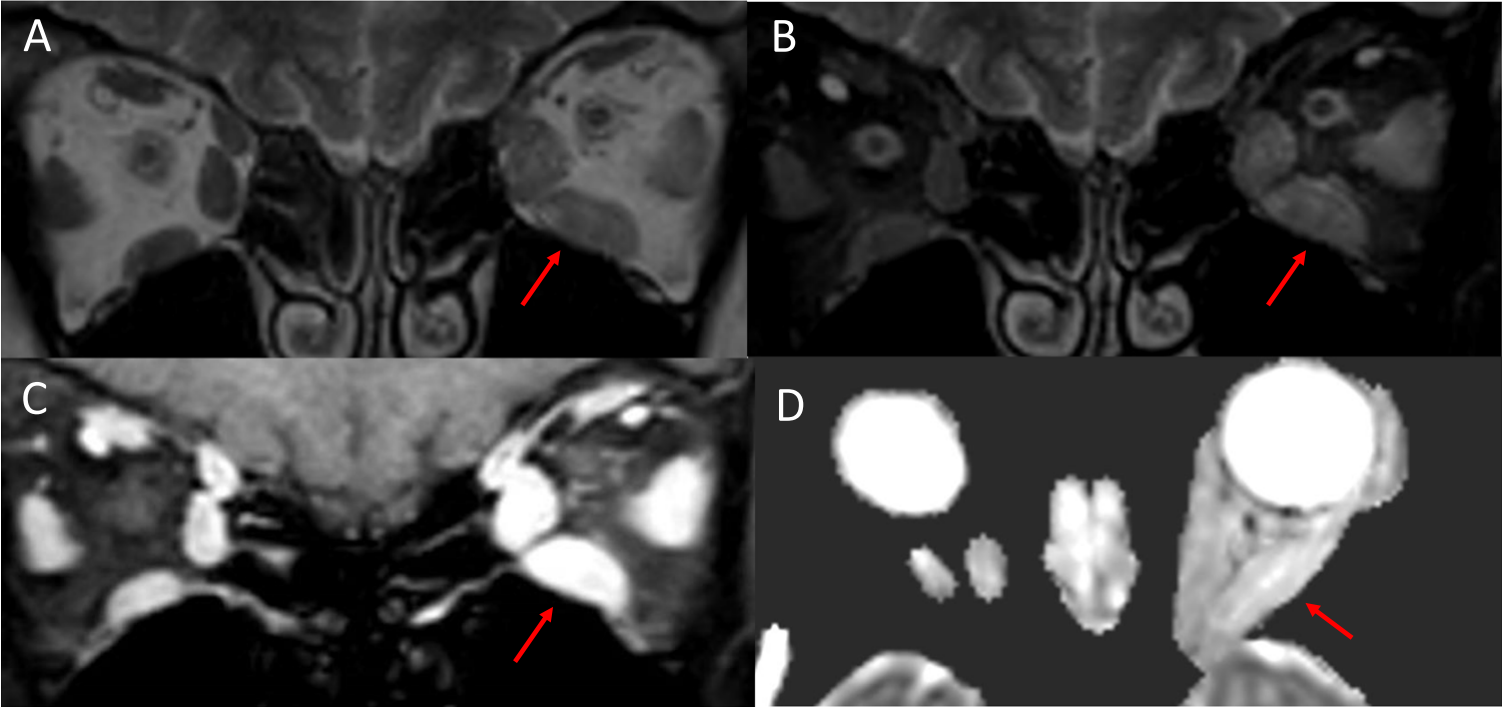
Active thyroid eye disease. Coronal T2-weighted MRI (A) showing enlargement and hyperintensity within the left inferior rectus, medial rectus and lateral rectus muscles (arrow). Coronal fat-suppressed T2-weighted MRI (B) demonstrating enlargement and hyperintensity of the left inferior rectus, medial rectus and lateral rectus (arrow). Coronal fat-suppressed T1-weighted MRI (C) showing relatively increased enhancement of the affected left extraocular muscles (arrow). ADC map (D) demonstrating a hyperintense signal within the left orbit (arrow)
